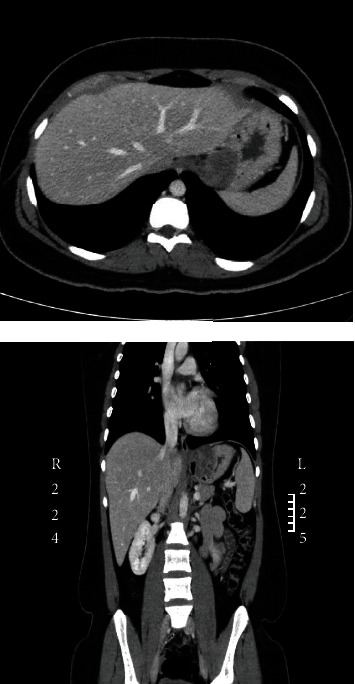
CT images showing a gastric mass. A 4.7 cm lobulated submucosal gastric mass at the gastric cardia near the gastroesophageal junction. The mass has an exophytic component abutting the left hemidiaphragm and left liver lobe.
